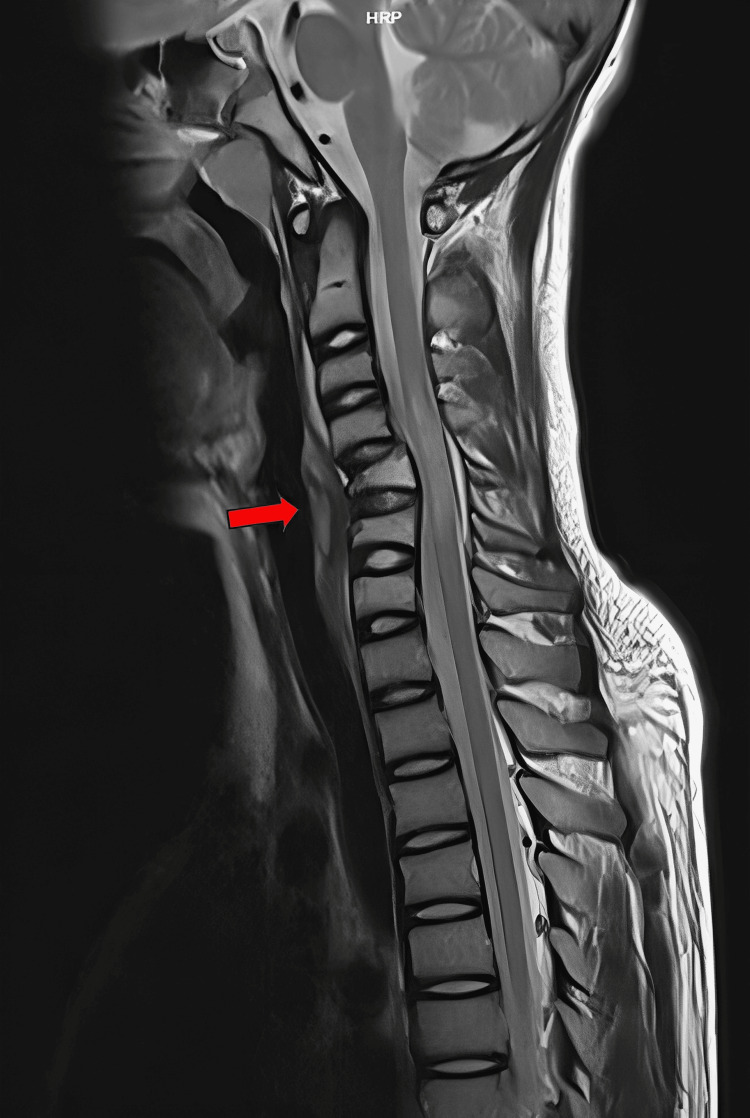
Sagittal view of cervical MRI with red arrow showing anterior dislocation of C4/C5 with bilateral perched facet joint and spinal cord oedema at the level of C4-C6 with intramedullary haemorrhage. MRI: magnetic resonance imaging
